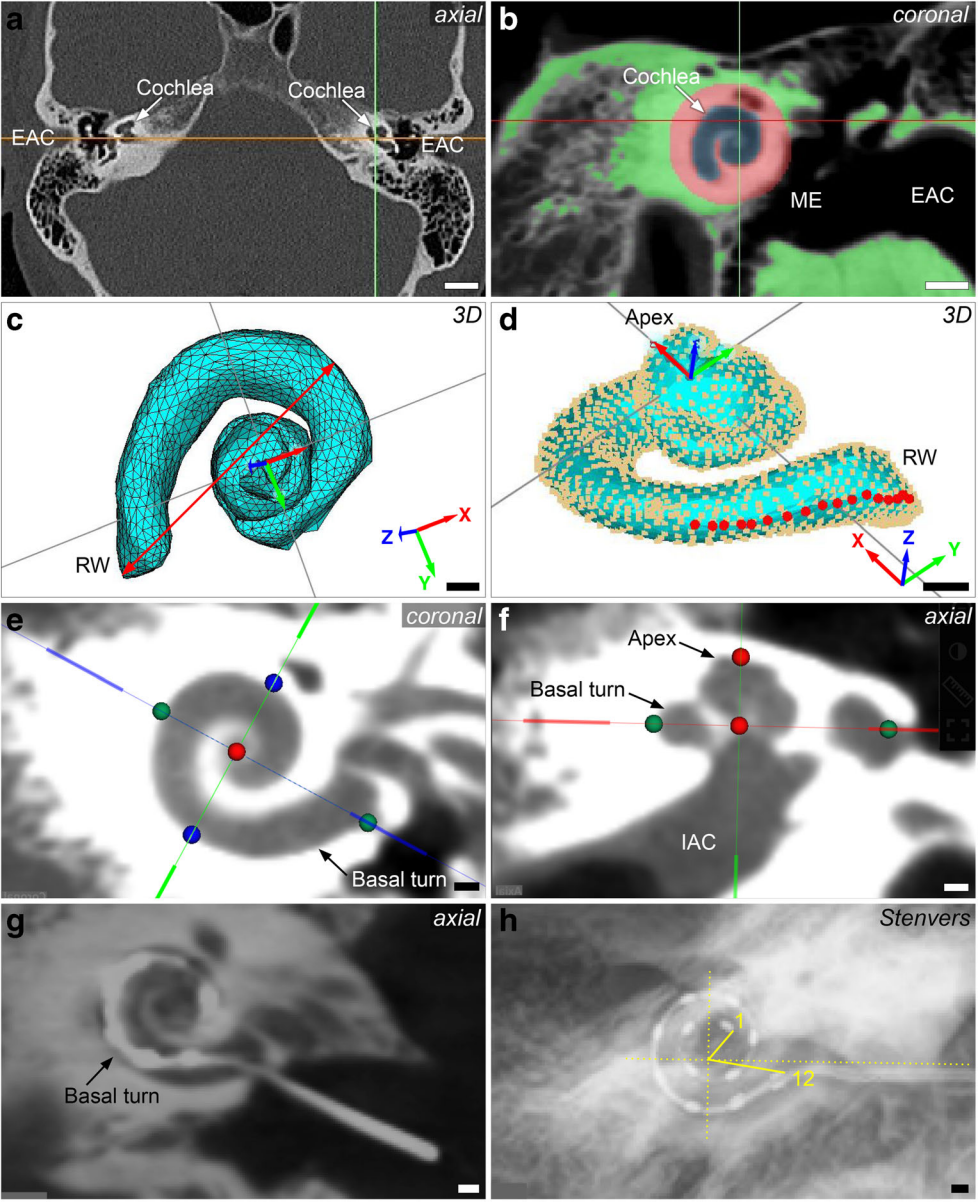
Cochlear duct length (CDL) measurement approaches. a Axial view of a high-resolution computed tomography (HRCT) scan in Materialise Mimics software. b Segmentation of a cone beam computed tomography (CBCT) scan in Materialise Mimics, threshold mask (green), region A (blue, cochlear fluid), and region B (red, otic capsule). c 3D model of the cochlea after segmentation. Red double arrow, measurement of the A-value from the round window (RW) to the opposite lateral wall (LW). Alignment: z-axis (blue), x-axis (red), y-axis (green) with z-axis pointing through the modiolus and the basal turn positioned in the xy-level. d Spline curve reconstruction from cloud point surface of the 3D model. Red dots: selected points on the LW. Alignment: z-axis (blue), x-axis (red) y-axis (green) with z-axis pointing through the modiolus and the basal turn positioned in the xy-level. e CBCT scan with view on the cochlea in the oblique coronal view in the otosurgical planning software, with measurements of the A-value (cochlear diameter; green dots) and the B-value (cochlear width; blue dots). f CBCT scan, with view on the cochlea in the axial view in the otosurgical planning software, red dots: determination of the height of the cochlea. g Postinsertion CBCT scan with view on the cochlea in the oblique coronal view in the otosurgical planning software showing the fully inserted electrode in the basal turn. h Postinsertion radiograph (Stenvers projection) showing the measurement of the insertion angle determined between the first (1.) and the twelfth (12.) electrodes. Yellow lines, angle of the first/twelfth electrode. EAC, external ear canal; IAC, internal auditory canal; ME, middle ear; RW, round window. Scale bars: 10 mm (a, b), 1 mm (c–h)
